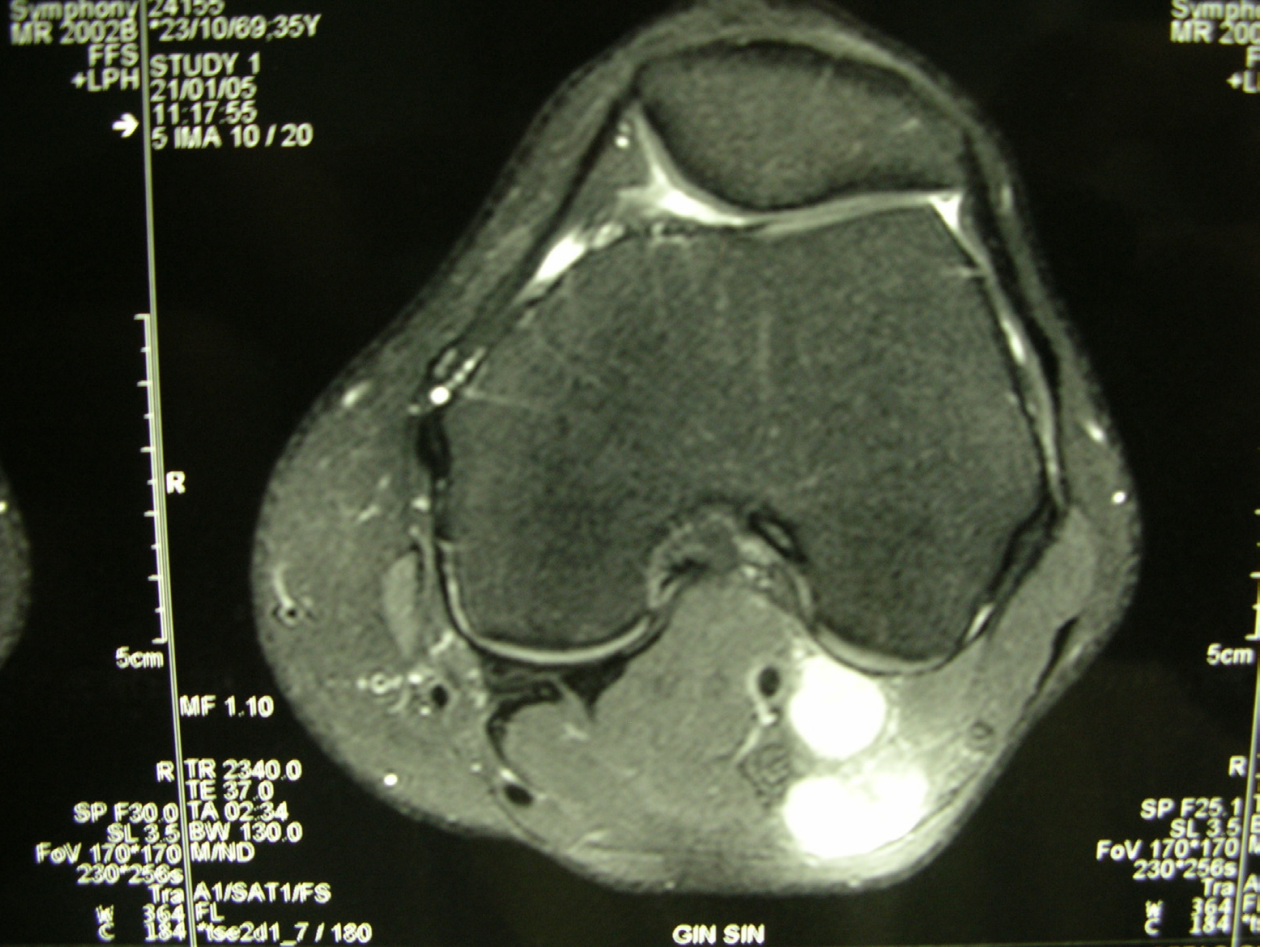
Axial MRI of the left popliteal fossa showing two enlarged nodes in close proximity to the popliteal vessels.
